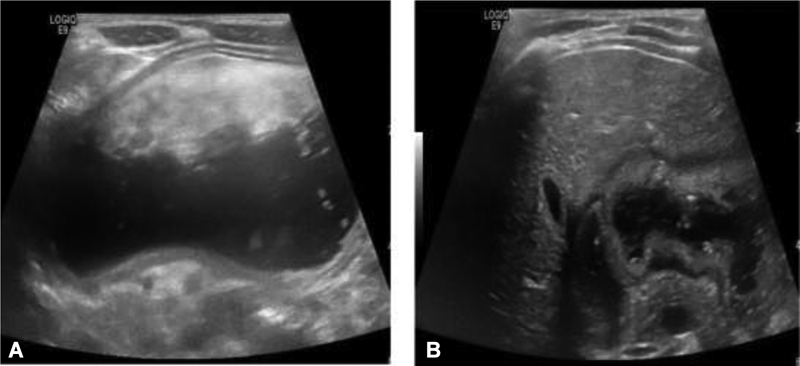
Ultrasound of the abdomen showing a grossly distended stomach. (
